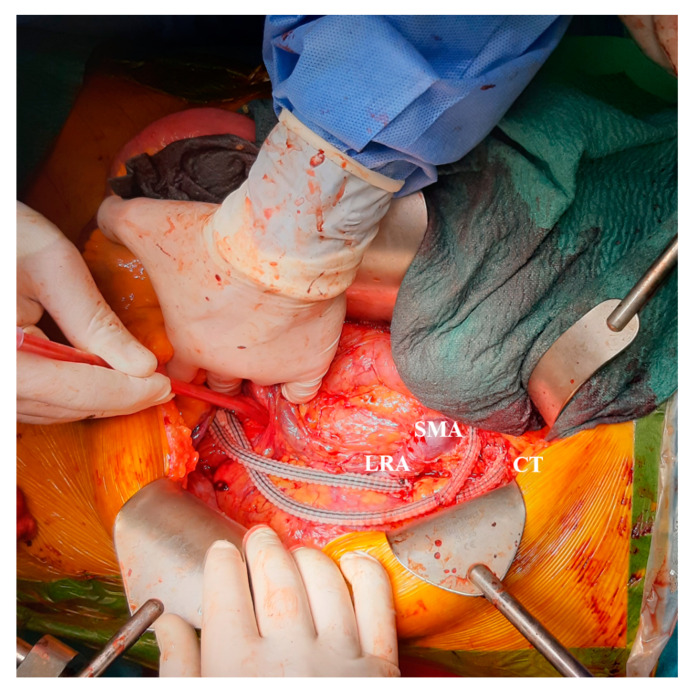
Intraoperative image showing the visceral debranching graft with its three branches supplying the left renal artery (LRA), the superior mesenteric artery (SMA), and the celiac trunk (CT).
